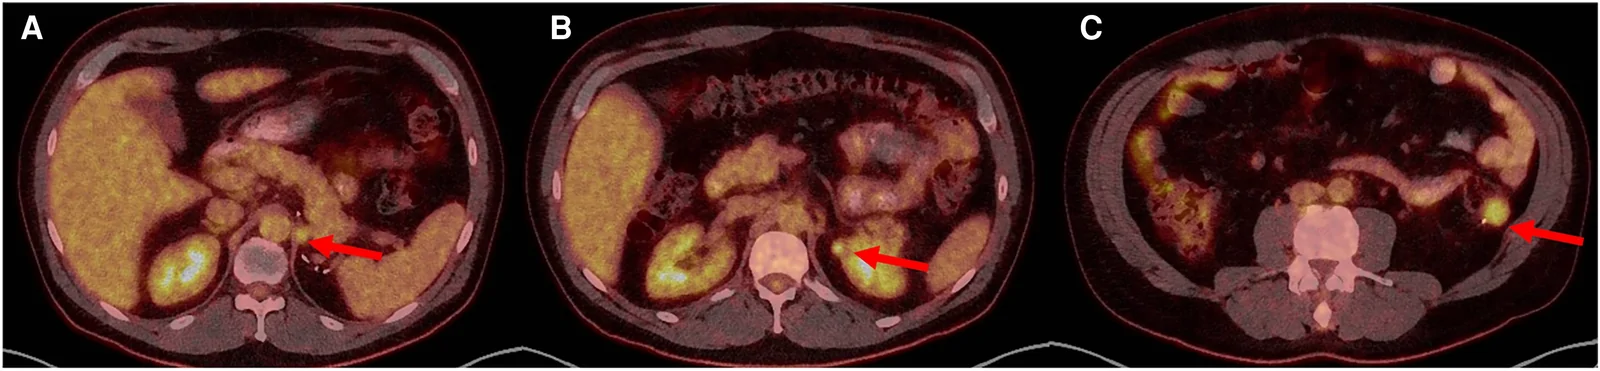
^18^F-fluorodeoxyglucose positron emission tomography (FDG-PET) demonstrating 3 recurrent adrenocortical carcinoma lesions (red arrows) in the left retroperitoneum, including (A) a nodule within the prior suprarenal surgical bed, (B) a nodule adjacent to the left kidney, and (C) a mass inseparable from the descending colon within the left paracolic gutter.
